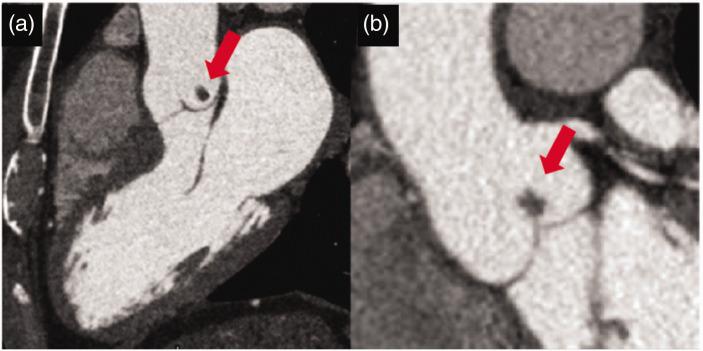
Cardiac computed tomography images showing a small homogeneous non-contrast-enhanced mass on the aortic side of the left coronary cusp in association with a papillary fibroelastoma (red arrows). (a) Long-axis view of the three chambers and (b) Long-axis view of the aorta (used with permission).
